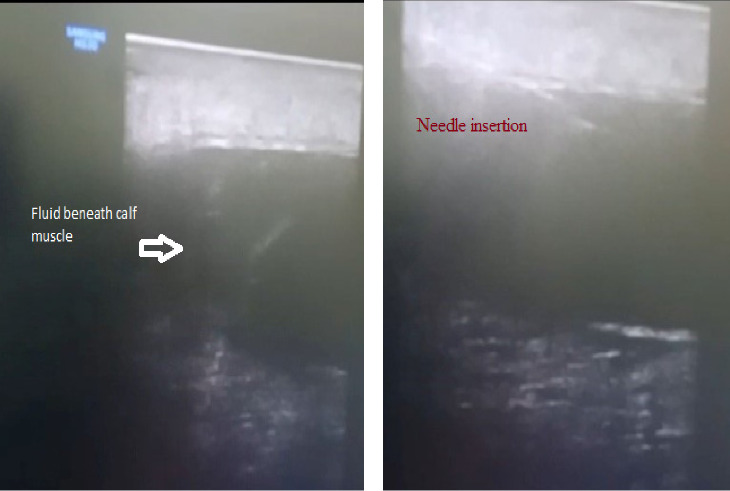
(a) Musculoskeletal ultrasound of the affected region showing large hypoechoic (with minimal hyperechoic) shadows resembling fluid (with debris) beneath muscle. (b) Ultrasound-guided needle insertion to aspirate fluid.
